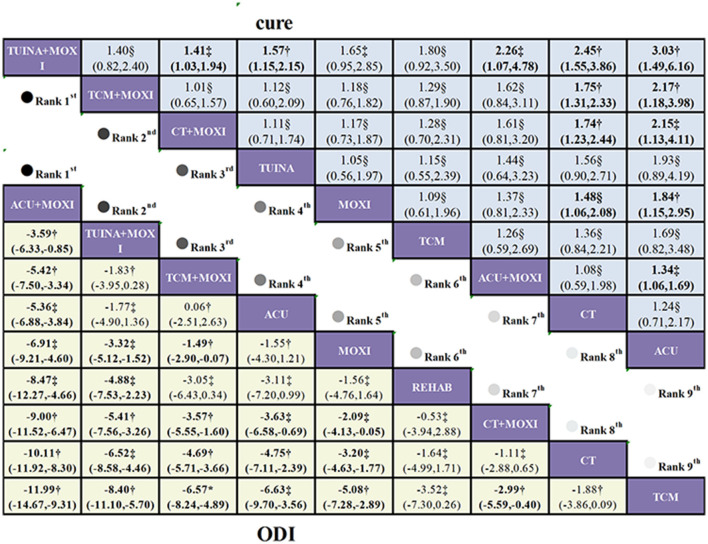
League table of treatment comparisons in the primary analysis of LDH. League table of the NMA for the comparative effectiveness of moxibustion-based combination therapies for lumbar disc herniation (ranked according to SUCRA values; outcomes: ODI score and cure rate). Interventions are ordered according to their SUCRA rankings (from highest to lowest probability of being the best treatment). Comparisons should be read from left to right. Comparative estimates are presented at the intersection between the treatment defined by the column and the treatment defined by the row. Continuous outcomes (ODI score) are reported as MDs, and dichotomous outcomes (cure rate) are reported as risk ratios (RRs), each with corresponding 95% CIs. Lower-left triangle (ODI score): MD < 0 favors the treatment defined by the column (greater improvement in functional disability).Upper-right triangle (cure rate): RR > 1 favors the treatment defined by the column. Bold numbers indicate statistically significant differences (95% CI does not cross 0 for MD or 1 for RR). CT serves as the reference comparator within the network; all relative effects are interpreted according to the treatments specified by the corresponding row and column. Certainty of the evidence for the two primary outcomes, assessed using the GRADE approach. *High certainty of evidence; ^†^moderate certainty of evidence; ^‡^low certainty of evidence; ^§^very low certainty of evidence.
